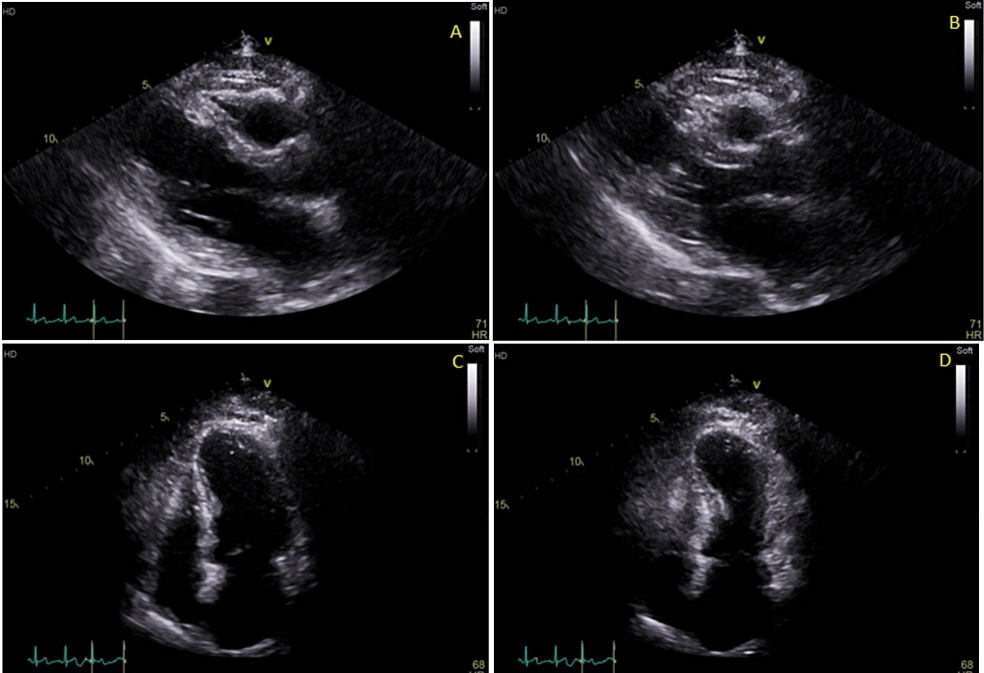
The patient’s transthoracic echocardiogram Echocardiogram images show apical akinesis and contraction of the mid and proximal portion of the left ventricle; (A) parasternal long axis at end-diastole (B) parasternal long axis at end-systole (C) apical four-chamber at end-diastole (D) apical four-chamber at end-systole.
